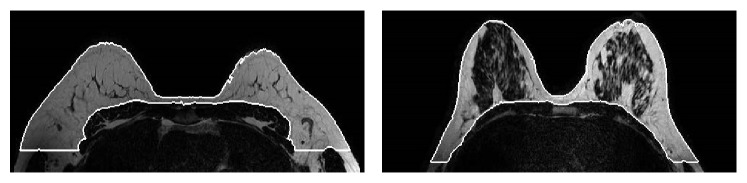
Multistate CNN used to segment small fatty breast and medium dense breast for MRI image [39].
